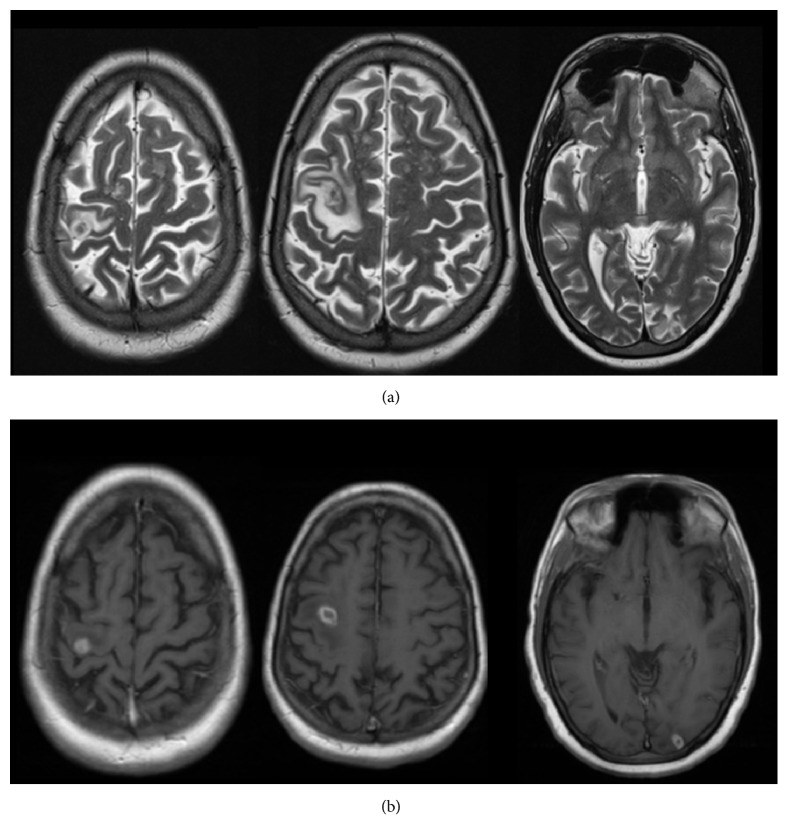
Brain MRI. (a) Axial T2 weighted: three nodular lesions, two in right frontal lobe and one in the left occipital lobe, with hypointense central core, hyperintense peripheral margin, and vasogenic oedema. (b) Axial T1 + gadolinium: ring-enhancing lesions and absent leptomeningeal enhancement.
